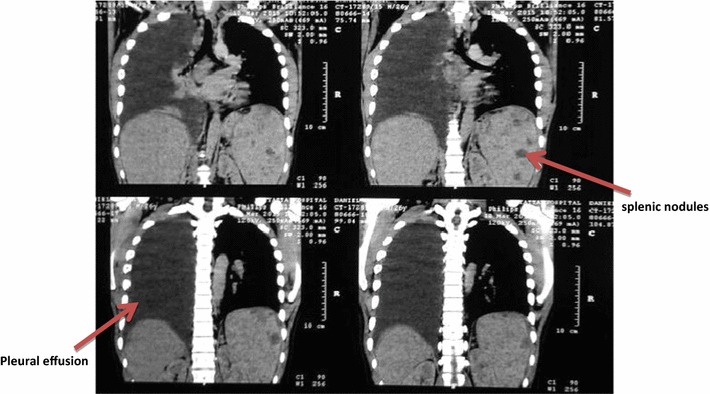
Chest computed tomography slices showing massive right pleural effusion and multiple splenic nodules
